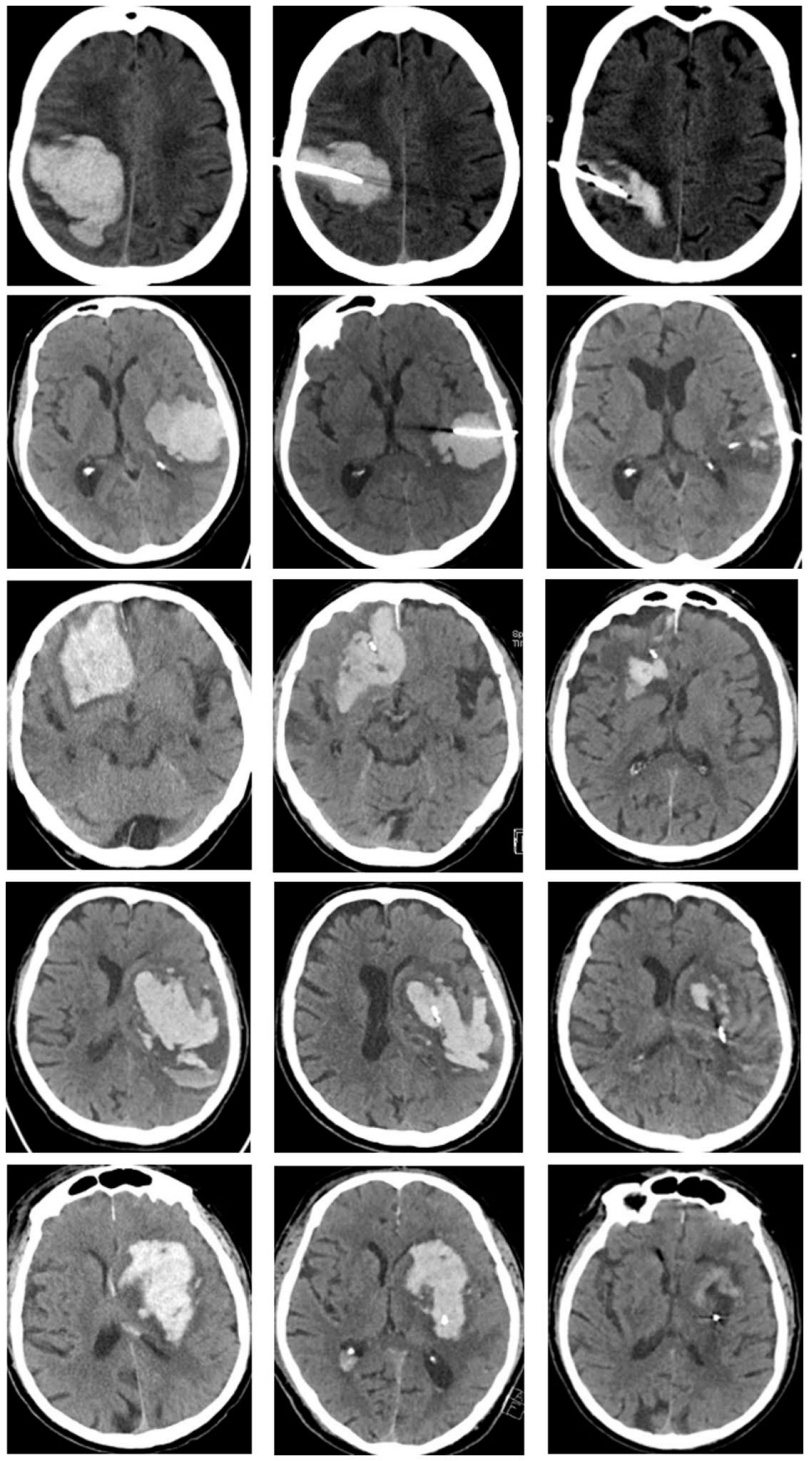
CT scans of differently located lobar ICH (row 1: occipital, row 2: temporal, row 3: frontal) and ganglionic ICH (row 4: posterior ganglionic, row 5: anterior ganglionic). CT images at admission (first column), immediately after catheter placement (second column), and at day 4 after completion of local lysis (last column).
